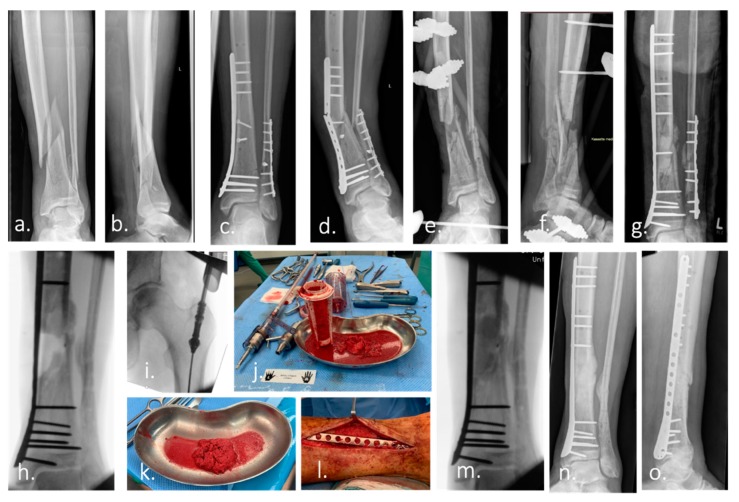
Case example of infected non-union treated with Masquelet technique and Reamer–irrigator–aspirator. (a,b) Ap and lateral radiograph of a multi-fragmentary Gustilo–Anderson Type II open tibia and fibula fracture of a 74-year-old man. (c) Ap radiograph after surgical treatment. (d) 7 month after surgery: re-presentation of the patient with an infected non-union of the tibia with re-fracture and failure of the osteosynthesis material. (e,f) Postoperative Radiograph of the lower leg (ap and lateral): Condition after removal of the broken osteosynthesis material. Large, bone defect of the tibia after extensive debridement, reaming of the medullary canal, radical resection of the non-union and temporary immobilization with an external fixator. The microbiological samples taken intraoperatively revealed the presence of Staphylococcus caprae. Antibiotic therapy was carried out according to the recommendations of the interdisciplinary infection board. (g) Re-osteosynthesis of tibia and fibula after resolution of infection. Temporary treatment of the bone defect with a cement spacer to induce a vascularized foreign-body membrane (Masquelet membrane). (h) Intraoperative radiograph after removal of the cement spacer 2 months after implantation. (i–k) Harvest of autologous bone graft (bone marrow, morselized bone) from the femur using the Reamer–irrigator–aspirator (RIA). (l,m) Picture and intraoperative radiograph after filling of the bone defect with autologous bone. (n,o) Ap and lateral radiograph of the left lower leg demonstrate healing of the bone defect 4 month after surgery.
